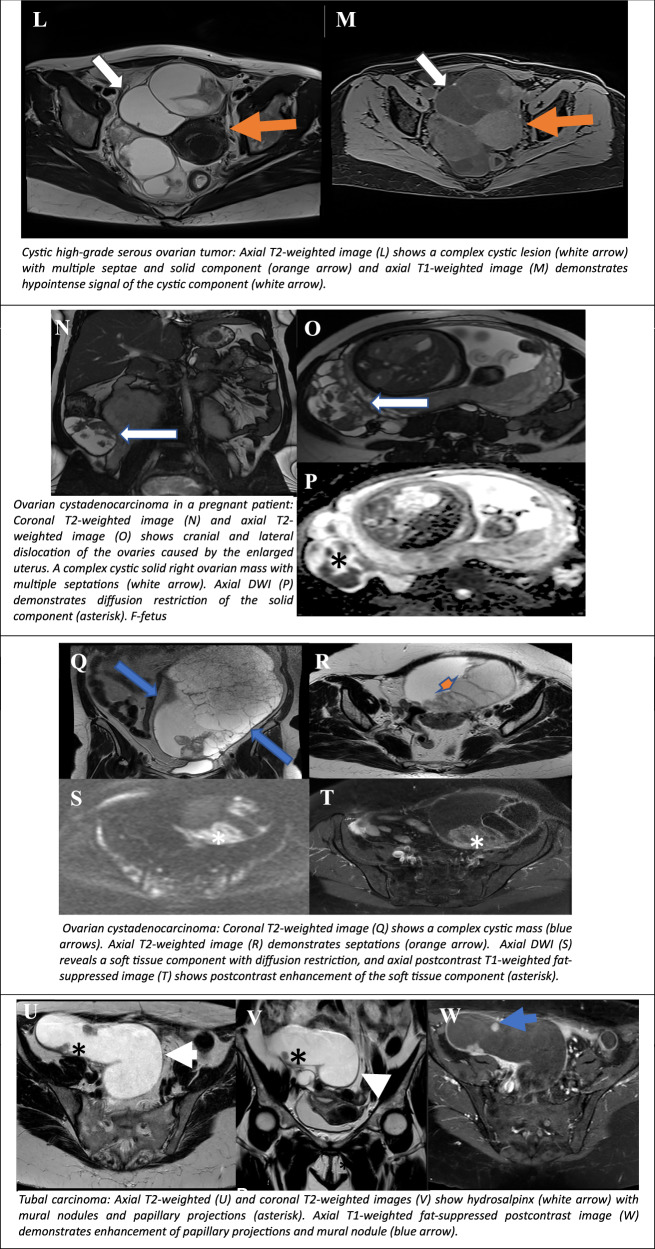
O-RADS MRI 5: cystic ovarian lesions with high risk for malignancy. Colon cancer metastasis in a patient with a history of hysterectomy and left adnexectomy 25 years ago for an unknown cause, and colon cancer 5 years ago. Routine control CT revealed a right adnexal mass. Axial T1-weighted image (A) shows a cystic right adnexal mass with mural nodules (orange arrows), which demonstrate restricted diffusion on high b-value (b:1000) DWI (B) and ADC map (C). Haemorrhagic metastasis of ductal breast carcinoma to the left ovary. In a patient with a history of hysterectomy and right adnexectomy for cervical carcinoma. Axial T2WI (D) shows a left-sided septated cystic-solid pelvic mass with equivocal origin (orange arrows). Axial DWI (E) demonstrates iliac lymphadenopathy. Axial T1-weighted fat-suppressed image (F) reveals haemorrhagic content within the mass (asterisk), and axial T1-weighted fat-suppressed postcontrast image (G) shows peripheral and septal enhancement. Ovarian metastasis of endometrial cancer: Axial T2WI (H) shows a hypointense endometrial mass (blue arrow) with more than 50% myometrial infiltration and extrauterine spread to the left ovary (white arrow). Axial DWI (I) demonstrates diffusion restriction of the ovarian mass (asterisk). T1-weighted fat-suppressed postcontrast image (J) reveals inhomogeneous enhancement of the endometrial and left ovarian mass (orange arrow). The dynamic contrast-enhanced (DCE) (K) curve shows a type 3 (malignant) pattern. Cystic high-grade serous ovarian tumour: Axial T2WI (L) shows a complex cystic lesion (white arrow) with multiple septae and a solid component (orange arrow), and axial T1-weighted image (M) demonstrates hypointense signal of the cystic component (white arrow). Ovarian cystadenocarcinoma in a pregnant patient: Coronal T2WI (N) and axial T2WI (O) show cranial and lateral dislocation of the ovaries caused by the enlarged uterus. A complex cystic solid right ovarian mass with multiple septations (white arrow). Axial DWI (P) demonstrates diffusion restriction of the solid component (asterisk). F-fetu. Ovarian cystadenocarcinoma: Coronal T2WI (Q) shows a complex cystic mass (blue arrows). Axial T2WI (R) demonstrates septations (orange arrow). Axial DWI (S) reveals a soft tissue component with diffusion restriction, and axial postcontrast T1-weighted fat-suppressed image (T) shows postcontrast enhancement of the soft tissue component (asterisk). Tubal carcinoma: Axial T2-weighted (U) and coronal T2WI (V) show hydrosalpinx (white arrow) with mural nodules and papillary projections (asterisk). Axial T1-weighted fat-suppressed postcontrast image (W) demonstrates enhancement of papillary projections and mural nodule (blue arrow)
